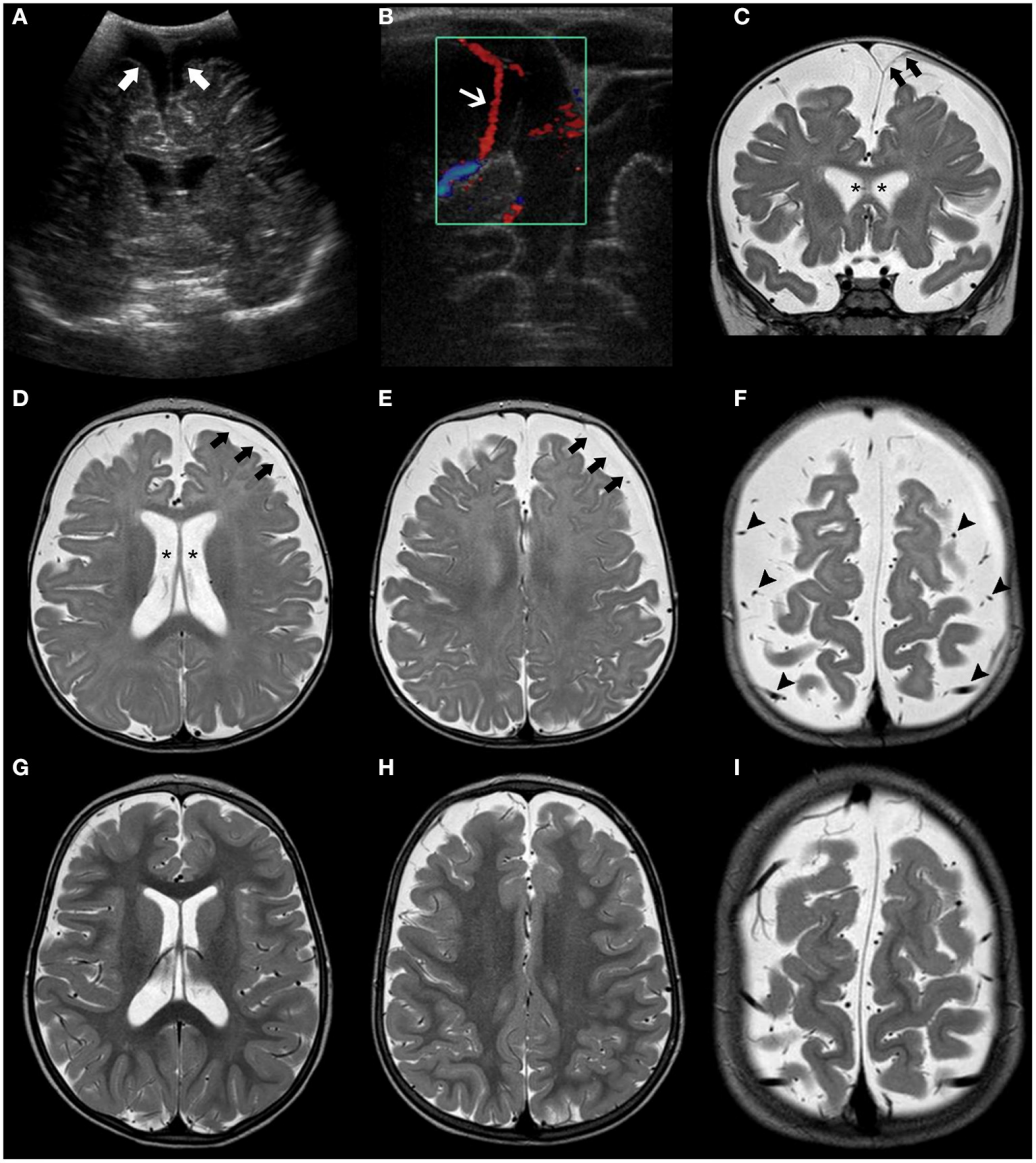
Brain MRI findings in Benign enlargement of subarachnoid spaces (BESS) of infancy. Coronal Head US image (A) of a 7-months-old girl with macrocephaly shows bilateral enlargement of the frontal subarachnoid spaces and anterior interhemispheric fissure (thick white arrows). Note the presence of a few vessels (white arrow) on US Doppler (B) crossing the enlarged subarachnoid space on the right side, corresponding to the “cortical vein sign.” Coronal (C) and axial (D–F) T2WI performed a few days after better depict these findings, including presence of multiple small vessels bilaterally within these enlarged subarachnoid spaces (black arrowheads). In addition, there is a small left frontal T2 hyperintense subdural collection (black thick arrows) as well as mild enlargement of the ventricular system (black asterisks). Follow-up brain MRI of the same patient performed at 2.3 years of age including axial T2WI (G–I) depict expected spontaneous interval reduction of the aforementioned enlarged subarachnoid spaces and resolution of the thin left subdural collection.
